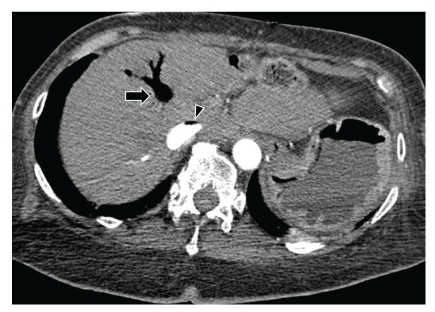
CT image of the liver shows air in the hepatic veins (*arrow*) and air-contrast level in the inferior vena cava (*arrowhead*).
